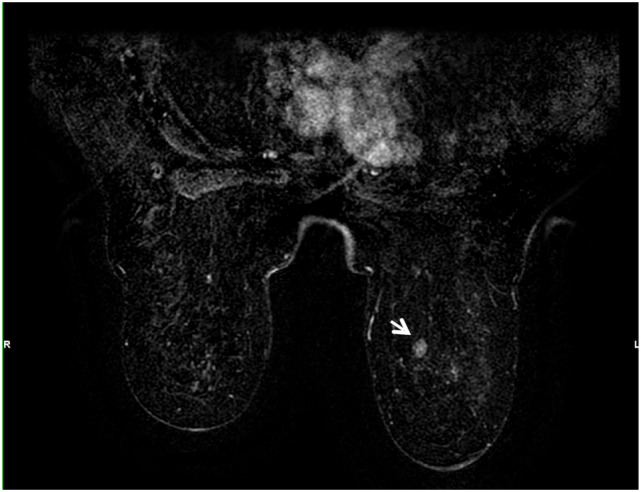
Gadolinium-enhanced T1-weighted images with fat saturation showing a remaining 1-cm focal enhancement 1 month after therapy (arrow).
